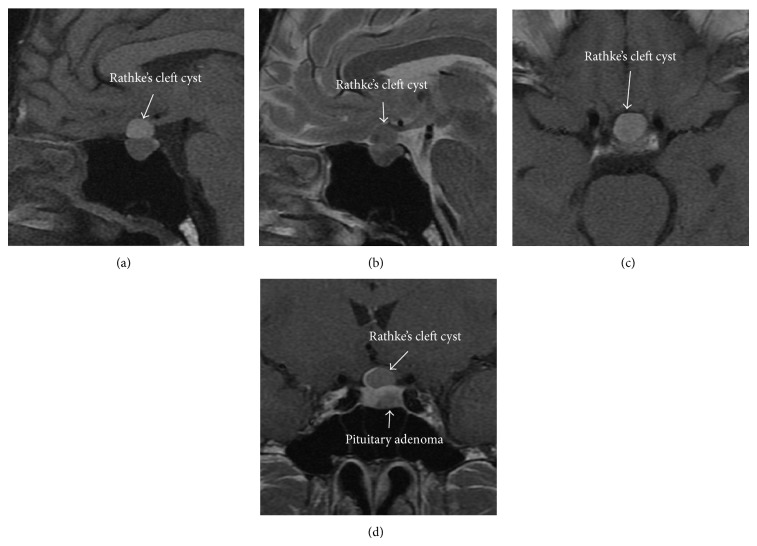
(a) Sagittal T1-weighted MRI of the head shows a suprasellar, high-intensity mass suspected to represent RCC. PA located below the RCC shows isointensity. (b) Sagittal T2-weighted MRI of the head shows isointense RCC and isointense PA. (c) Contrast-enhanced axial MRI shows nonenhancing RCC. In contrast, the normal pituitary gland shows strong enhancement. (d) Contrast-enhanced coronal MRI shows slight compression of the optic chiasma by RCC. And it shows an intrasellar PA of 9 mm in diameter located on the left of normal gland and suprasellar RCC of 12 mm in diameter that compressed stalk to the right side.
